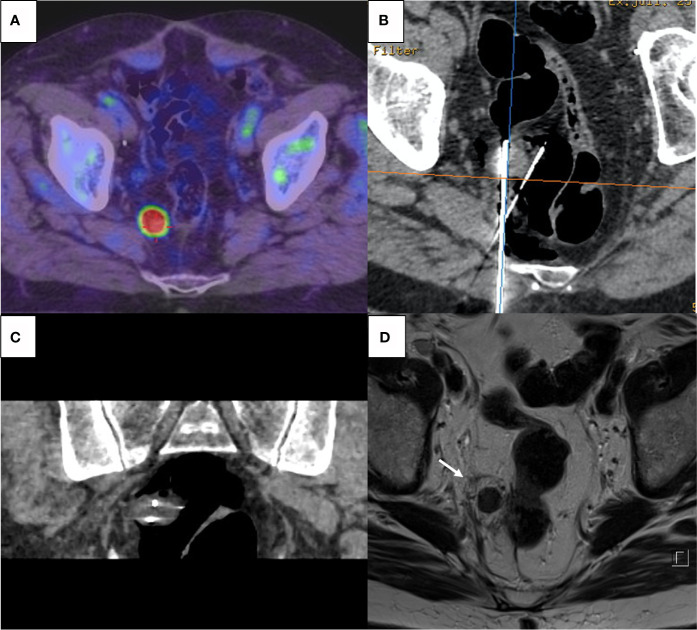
(A) Pre-procedure PET-Choline showing a 2 cm hypermetabolic lymph node abutting the rectal wall. (B, C) CT-guided percutaneous cryoablation with axial view (B) and coronal reconstruction (C); two cryoprobes were inserted into the target and CO_2_ insufflation *via a* 22-gauge spinal needle was performed to achieve rectal wall displacement and isolation. (D) T2-weighted MRI after 6 weeks showing complete necrosis of the nodule with surrounding halo of cytosteatonecrosis (arrow); absence of complication on the rectal wall.
